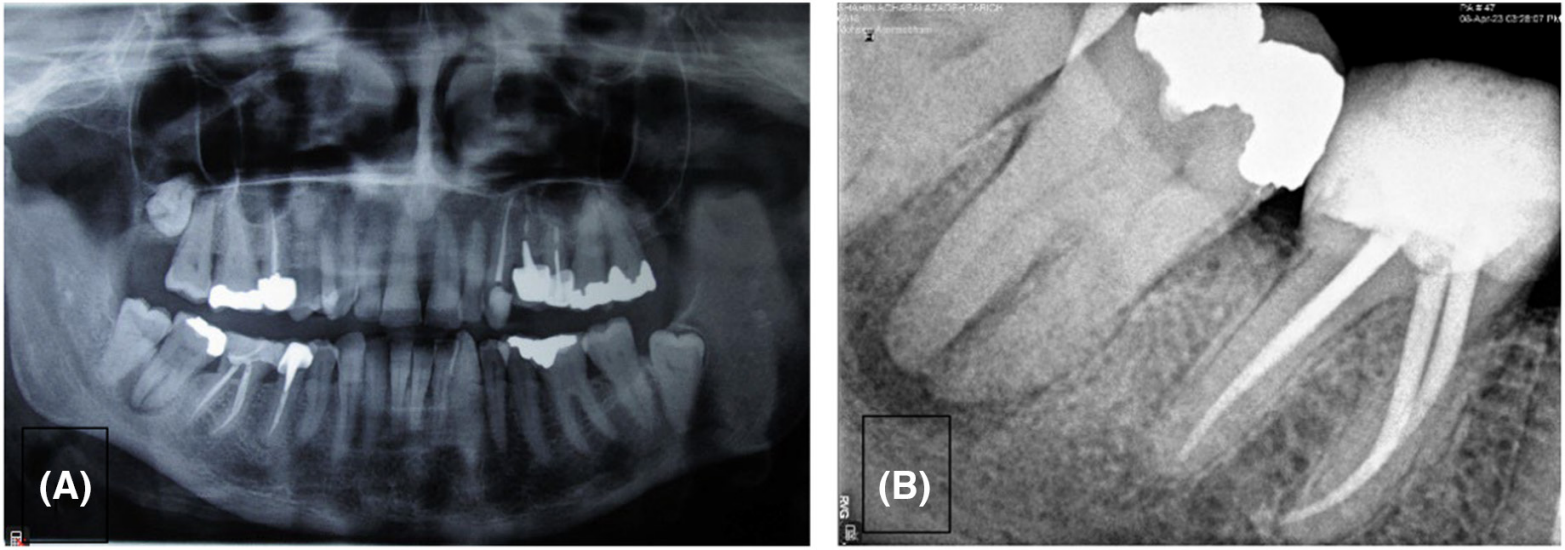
Panoramic view (A) and periapical radiograph (B) showing the periapical lesion.
